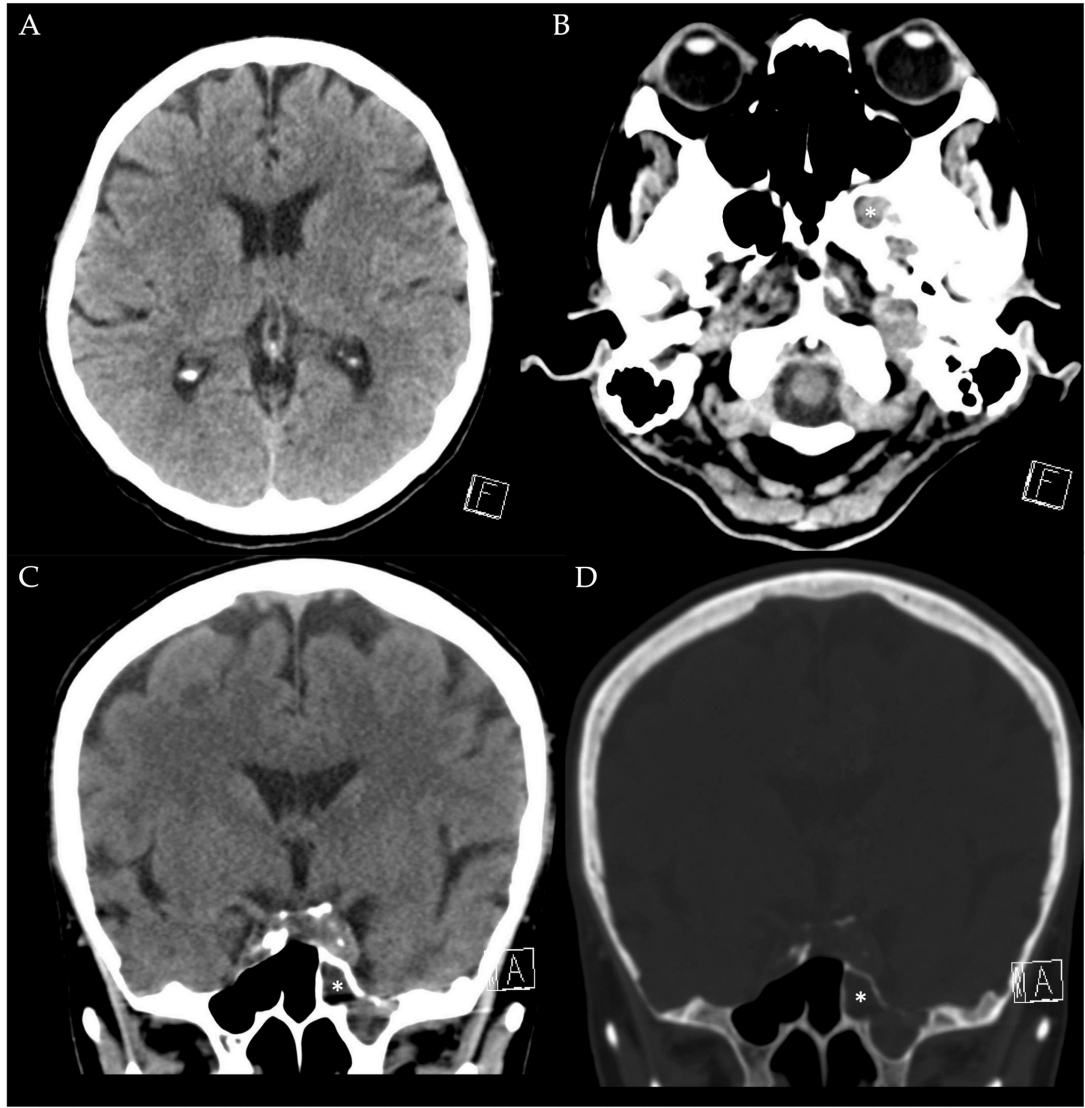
Non-contrast CT brain. 1A (Axial, soft tissue window), showing no significant intracranial abnormality on admission; 1B (axial, soft tissue window); 1C (coronal, soft tissue window); and 1D (coronal, bone window) showing sphenoid sinus opacification (white asterisk).
